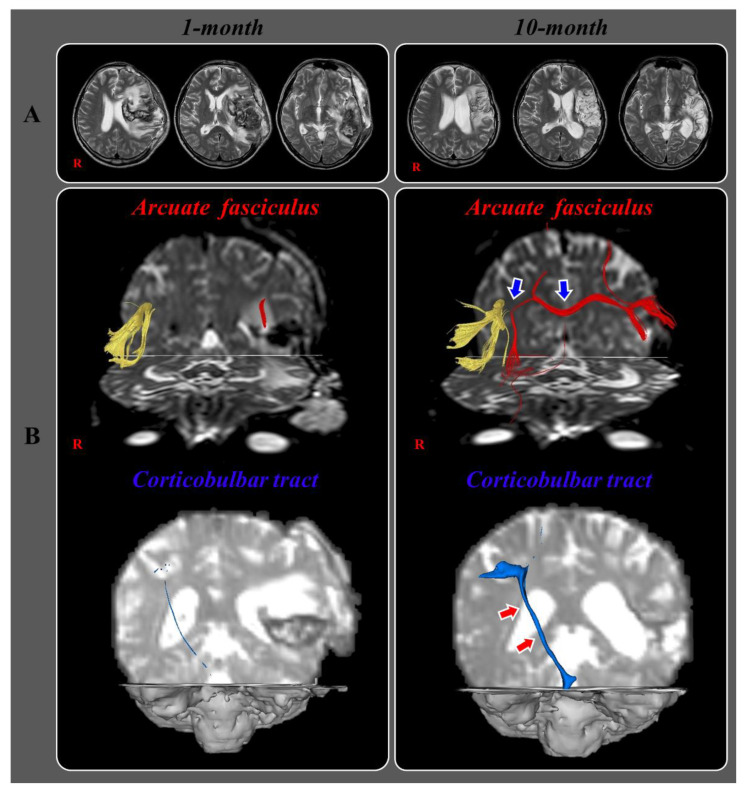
(A) Brain MR images at 1 month after onset show an infarct in the left middle cerebral artery territory, hemorrhagic transformation and subfalcine herniation. Brain MR images at 10 months after onset reveal leukomalactic lesions in the left fronto-parieto-temporo-occipital areas (R: right). (B) Results of diffusion tensor tractography (DTT) for the arcuate fasciculus (AF) and corticobulbar tract (CBT). On 1-month DTT, the discontinuation of the left AF and severe narrowing of the right CBT are observed. By contrast, on 10-month DTT, the left AF is connected to opposite AF by a new tract that passed through the splenium of corpus callosum (blue arrows) and the right CBT become thicker (red arrows) (reprinted with permission from Ref. [28]).
